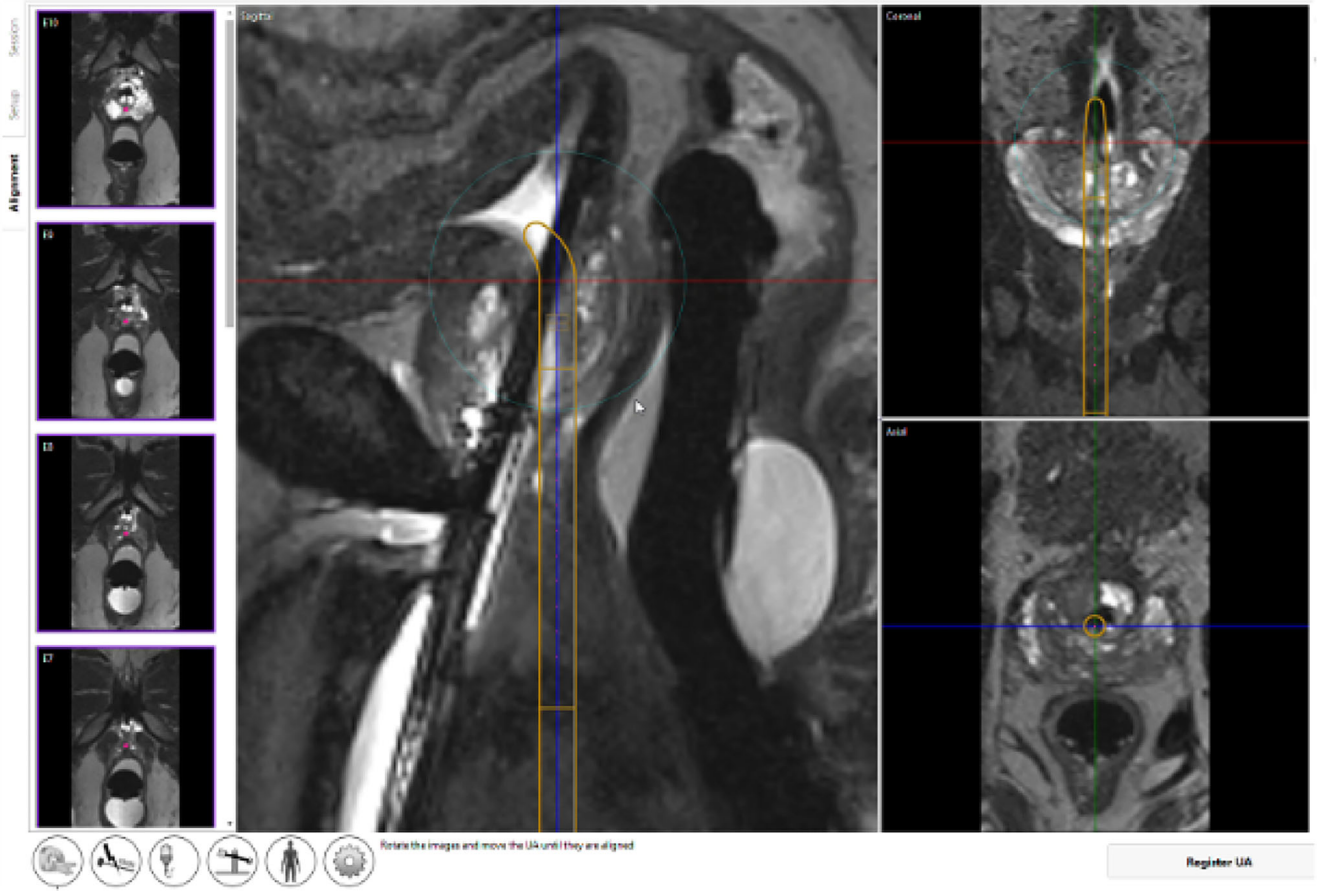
Device registration. The aim is to align the graphical representation of urethral applicator (orange line) with the actual physical position of urethral applicator on all three planes of MR images (represented by the cross hairs in red, blue and green lines). This allows subsequent ablation energy to be delivered accurately within the prostate
